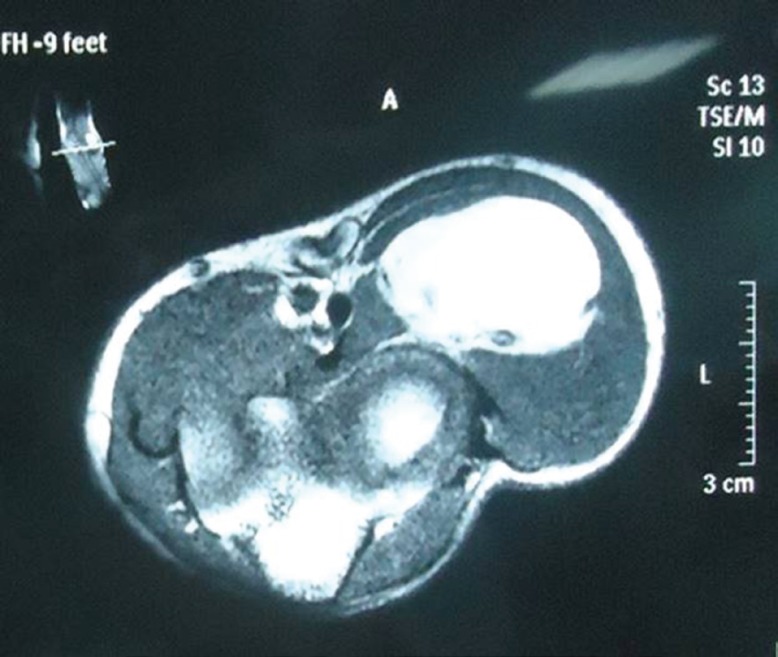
MRI (transverse section) showing relationship of the parosteal lipoma to the underlying radius and the adjacent muscles of the forearm
